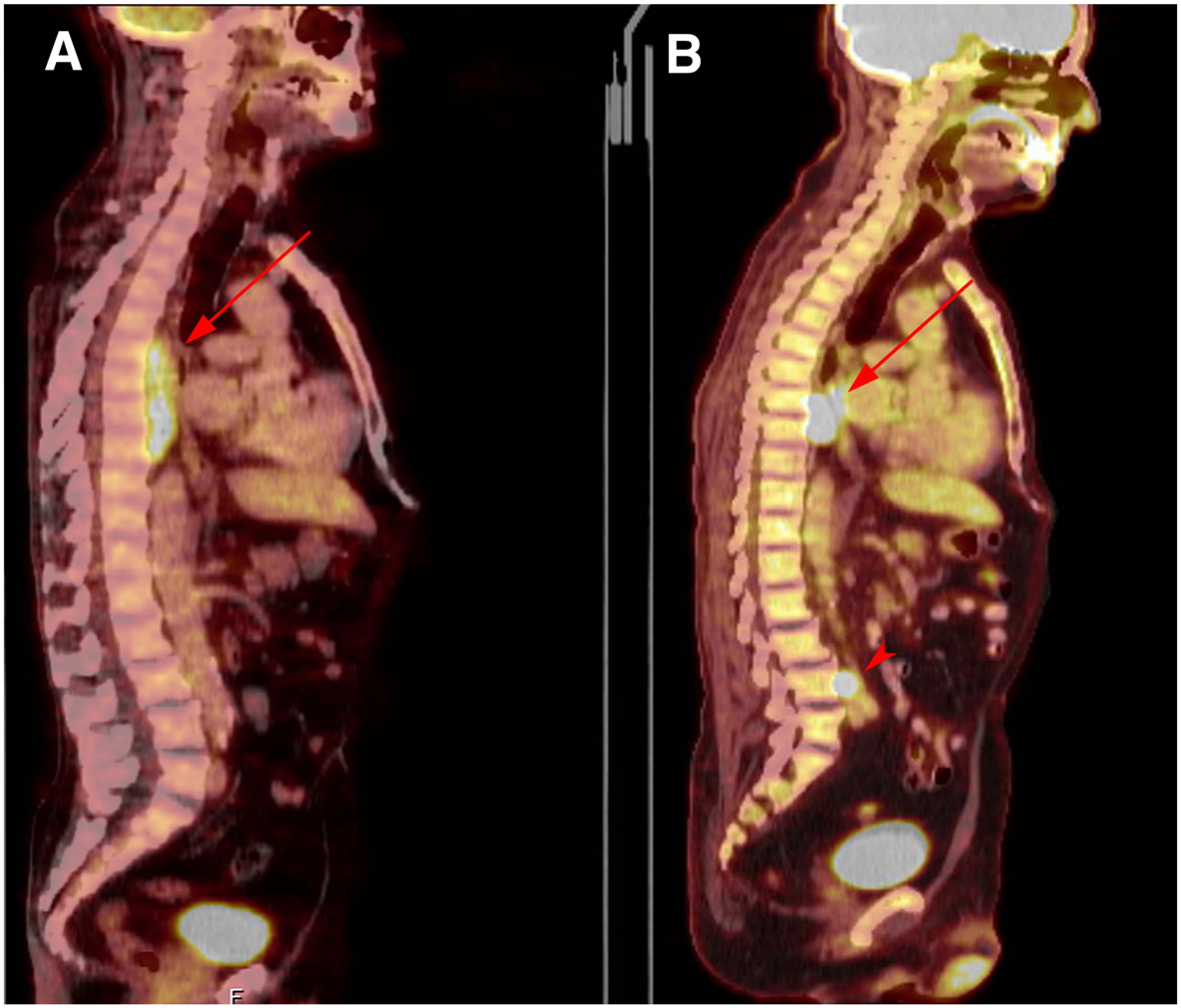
PET scan imaging before and after four weeks of Rituximab. a PET/CT scan before immunotherapy. Note a large metabolically active paravertebral mass of soft tissues adjacent to thoracic vertebral bodies T6 to T9 (arrow). A lesion in inter-aorto-caval mass is not visible at this level. b PET scan after immunotherapy; partial uptake reduction of the main thoracic paravertebral mass (arrow) and the inter-aorto-caval lesion (arrowhead)
